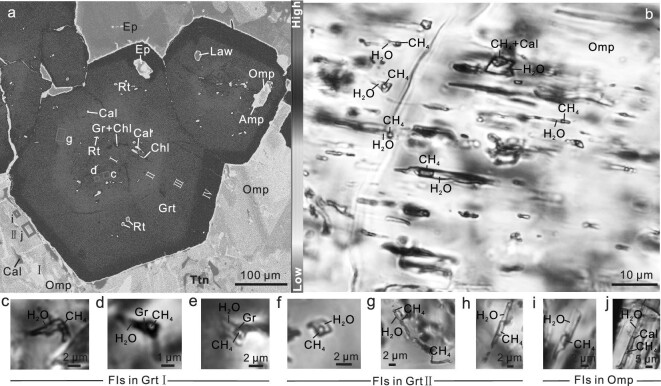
Examples of CH_4_-rich fluid inclusions in eclogite from the Western Tianshan subduction zone. (a) An Fe X-ray map showing the four well-preserved growth zones of garnet (Grt I–IV), abundant CH_4_-rich fluid inclusions preserved in Grt I and Grt II, and the paucity of fluid inclusions in Grt III and Grt IV. (b) Abundant tubular two- or three-phase CH_4_-rich fluid inclusions parallel with the long axis of omphacite (Omp). (c–j) Examples of two- or three-phase CH_4_-rich fluid inclusions in (c–e) Grt I, (f–h) Grt II and (i–j) Omp; gas phases are CH_4_ with minor N_2_, liquid phase is H_2_O, and solid phases (if present) are calcite (Cal) and graphite (Gr). Note that the representative locations of the CH_4_-rich fluid inclusions are marked in numbered squares in (a). Supplementary Figs S2–S6 provide additional element maps, microphotos and locations of CH_4_-rich fluid inclusions.
